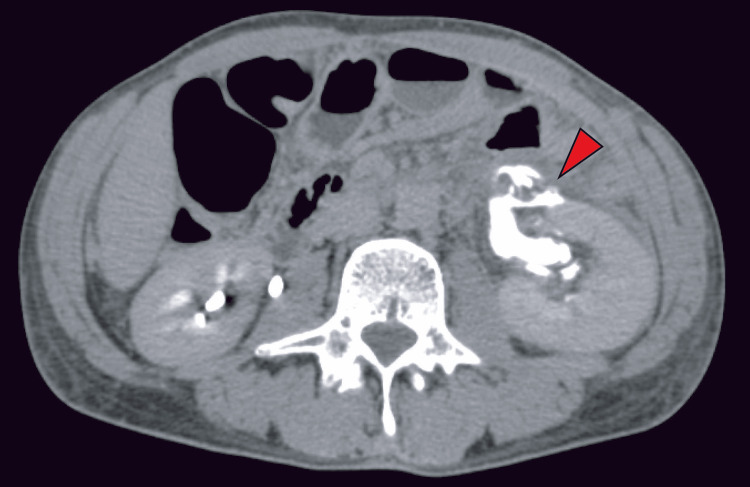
Delayed-phase contrast-enhanced abdominal CT on hospital day two Red arrows indicate the site of contrast medium extravasation. Delayed-phase contrast-enhanced CT demonstrated extravasation of contrast material from the left renal pelvis into the left perirenal space.
